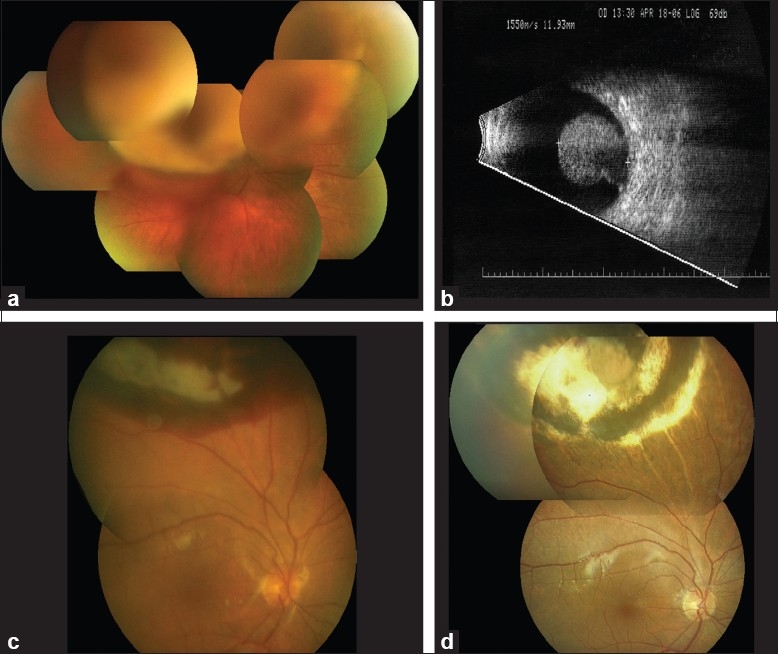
(a) Large choroidal melanoma obscuring funduscopic view to the posterior pole. Visual acuity is 20/100. (b) B-scan ultrasound of the tumor depicted in Figure 4(a) showing a 11.9-mm-thick mushroom-shaped choroidal melanoma. (c) Early postoperative view of the fundus of the eye depicted in (a) 2 days after endoresection. Visual acuity is 20/200. (d) Late postoperative view of the fundus of the eye depicted in (a) 18 months after endoresection. Visual acuity is 20/25.
